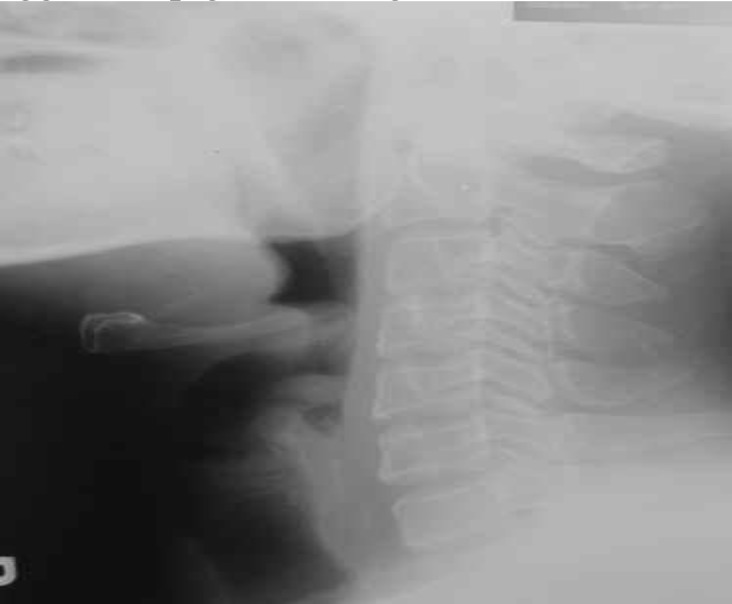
Radiography Shows the Thumb Sign
